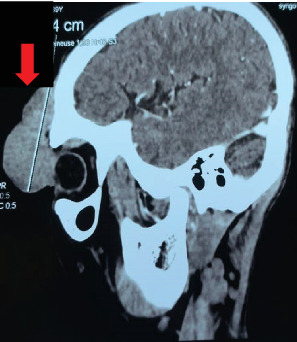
Contrast-enhanced head CT (sagittal view) showing a well-defined, enhancing soft tissue mass in the left frontal region, measuring 64 × 56 × 32 mm (red arrow). The lesion is associated with underlying bone lysis involving the anterior wall of the frontal sinus.
